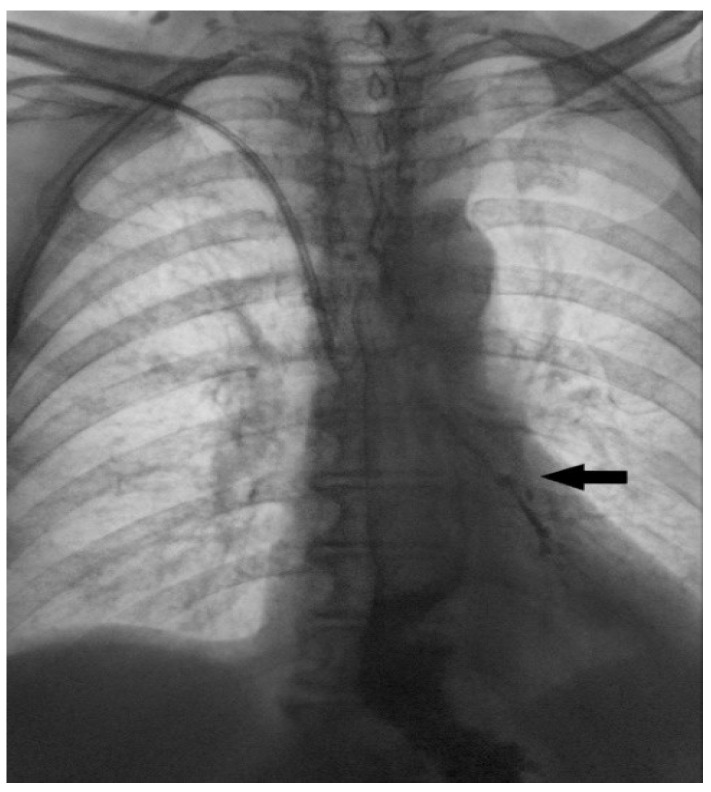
Contrast radiography is a very useful method in terms of establishing this diagnosis. The appearance of contrast in the lumen of the tracheobronchial tree (black arrow) is a direct sign of the fistulization of these structures within the esophagus. This image shows a clear esophagobronchial leak (fistula), which initiated a pronounced cough during the patient’s examination. Tracheobronchoscopy was also performed, showing an opening on the left side of tracheal bifurcation. In the further course of treatment, percutaneous endoscopic gastrostomy (PEG) was performed, and feeding via PEG was initiated. The patient responded well to antibiotics treatment, and was extubated 7 days after the admission to the intensive care unit. Surgery usually represents the primary treatment modality for acquired TEF. However, when surgery is contraindicated or cannot be performed as a primary solution, non-surgical treatment options may be explored. These can serve as a bridge to definitive surgical repair or, in cases of smaller fistulas, may even offer a definitive therapeutic option [3]. Esophageal stenting is an attractive, minimally invasive approach, used in treating predominantly malignant TEFs but also benign etiology [4]. Studies show a great decrease in respiratory complications, length of hospital stay, and overall quality of life, as well as better performance rate in these patients [5,6]. A retrospective study, comparing 22 patients with benign TEF treated with esophageal stenting showed successful closure of the fistula, obtained in 45% of cases. However, if the fistula is located in the proximal third of the esophagus, stenting may not be feasible [7]. Other authors utilize esophageal stenting together with airway stenting. This kind of approach may be more effective than esophageal stenting alone; however, the friction between the two stents can often damage the tissue between the trachea and esophagus. As a result, this combined approach is reserved only in selected cases [8]. After stabilizing the patient’s general condition and conducting a detailed case analysis, experts in this field decided to use a minimally invasive treatment option provided by interventional vascular radiology and attempt to close the fistulous tract by placing an IMPEDE Embolization plug coil to seal the opening in the wall of the esophagus and the trachea. In consultation with the interventional radiologist, the procedure was precisely planned. First, a flexible endoscope was introduced into the esophagus and a guidewire was placed from the lumen of the esophagus into the fistulous tract and a bronchoscope was used to confirm that the end of the guidewire was in the lumen of the trachea. Then, two IMPEDE Embolization plugs were successively placed one on top of the other, to fill the fistulous canal.
